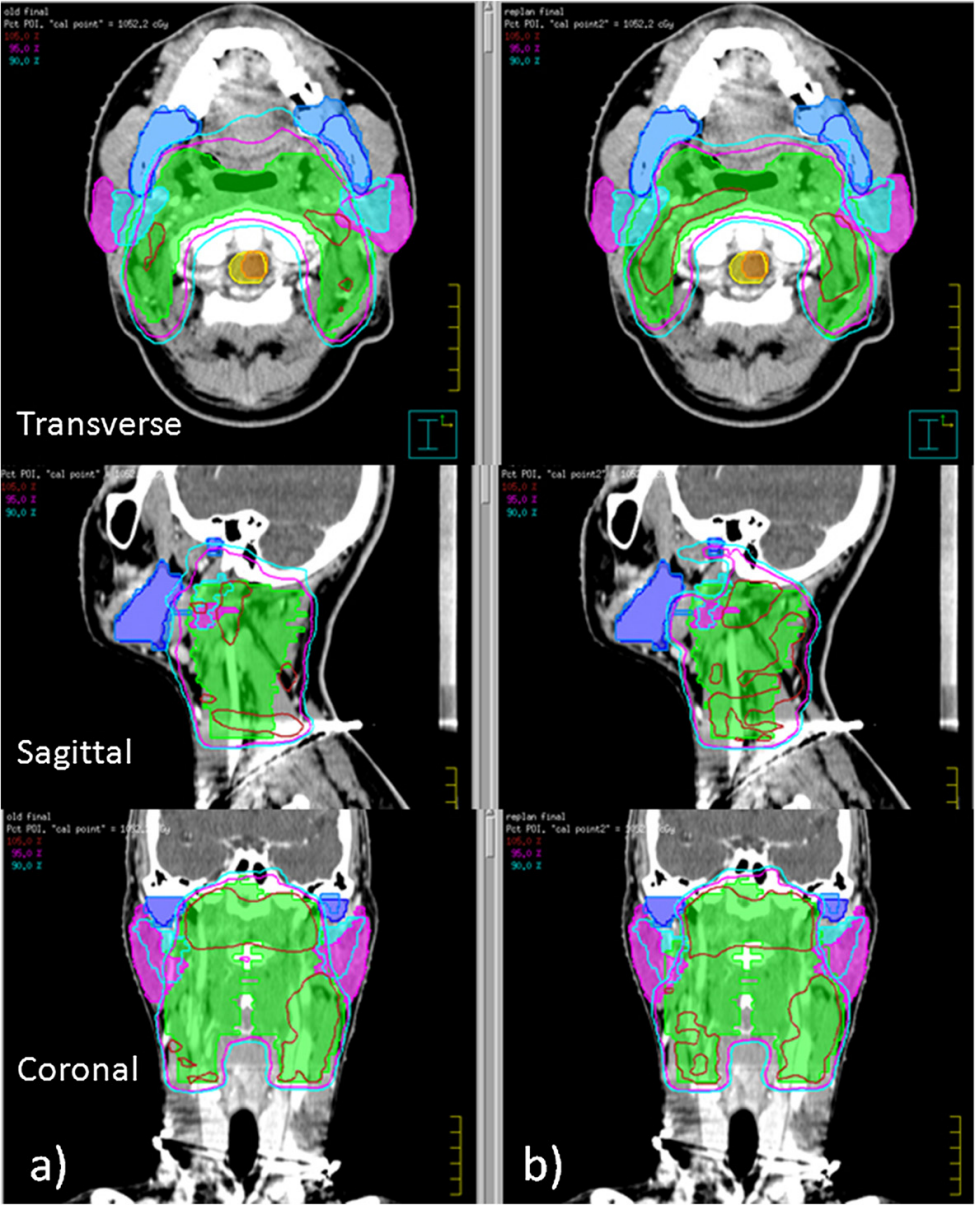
Typical dose distributions. A typical dose distribution of (a) the original VMAT plan delivered to the new contours projected in the initial planning CT, and (b) the re-optimized VMAT plan dose distribution based on the new contours projected in the iCT.
